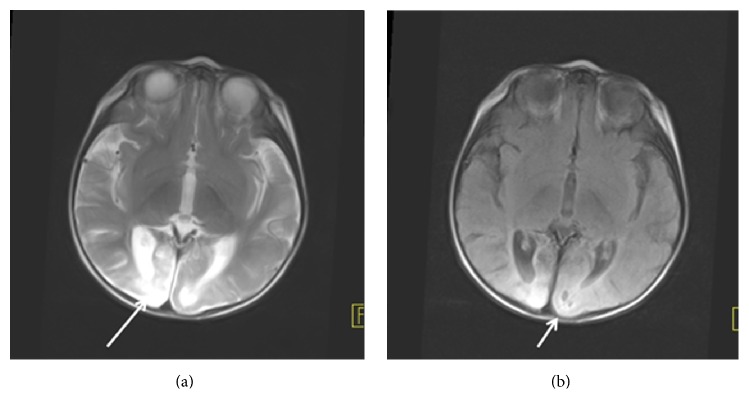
On brain Magnetic Resonance (MR) images, both visual cortices were hyperintense (a) (long arrow) on T2-weighted and flair weighted (b) (arrow) series.
